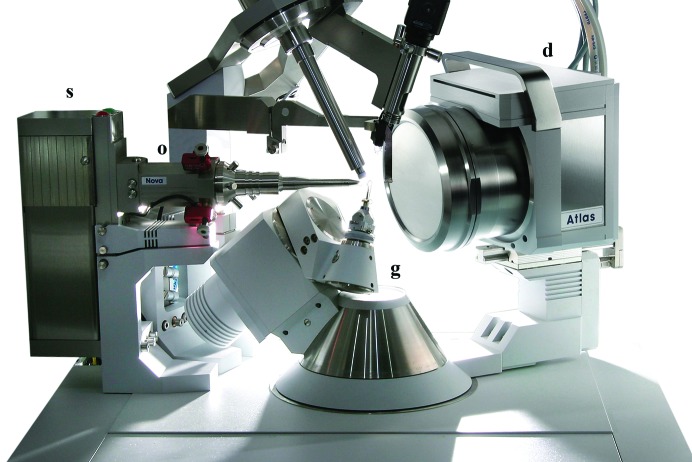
A compact X-ray system consisting of a microfocus sealed-tube source (s), focusing multi-layer optics (o), a four-circle goniometer (g) and a CCD detector (d). Picture courtesy of Agilent Technologies.
